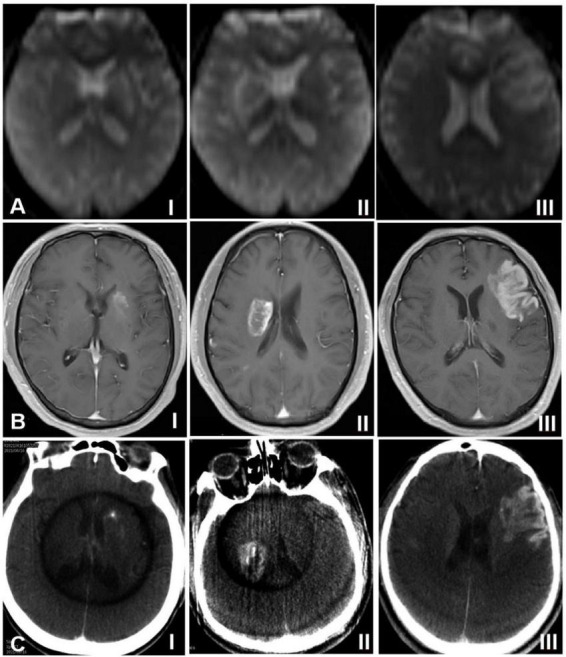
Classification of hemorrhagic transformation on arterial spin labeling imaging (ASL), dynamic contrast-enhanced (DCE) sequence of magnetic resonance imaging, and Xper CT. (A) ASL sequence imaging, (B) DCE sequence imaging, and (C) Xpert CT imaging. I indicates type I, II indicates type II, and III indicates type III.
